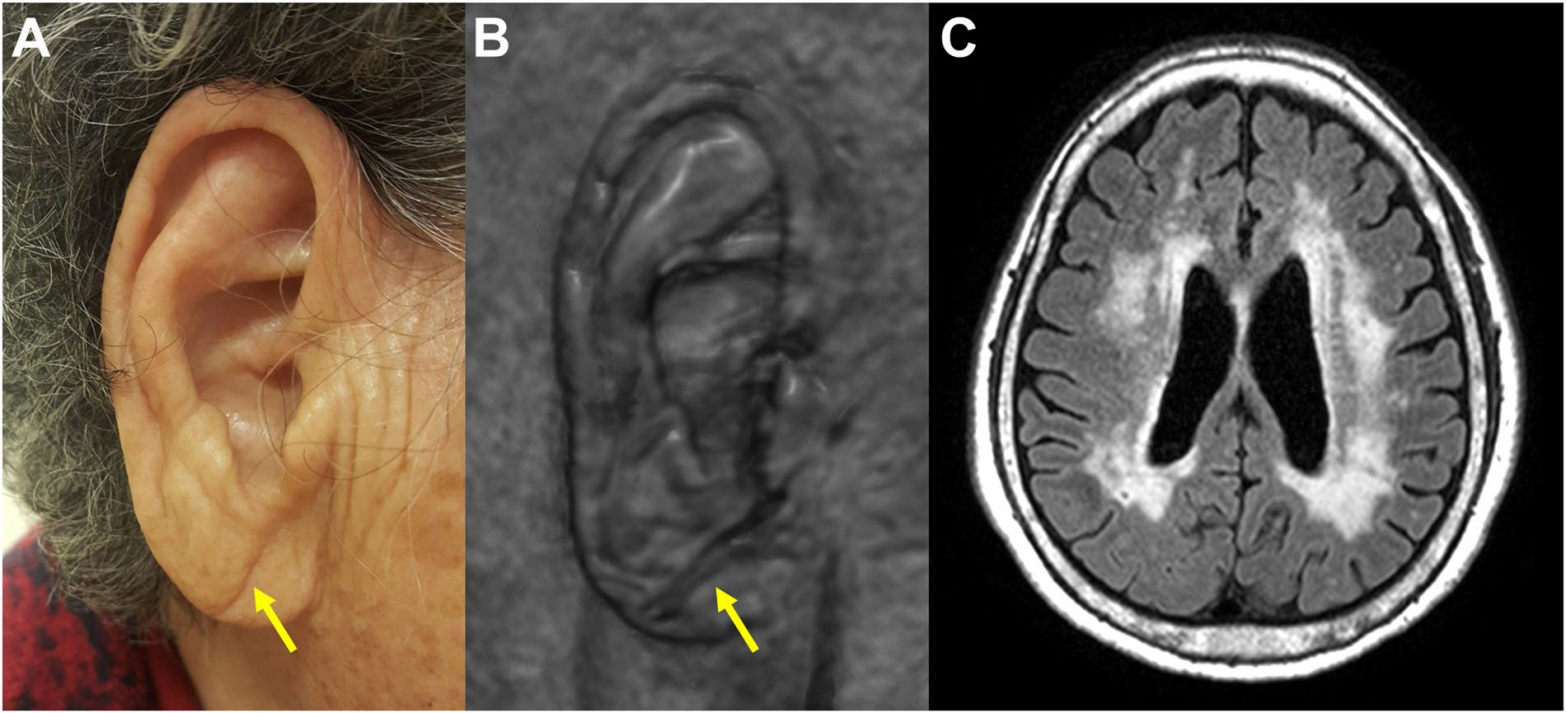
DELC (arrows) on photograph (A) and 3D-reconstructed MRI (B) in a cognitively impaired patient. The patient’s brain MRI shows severe WMH on FLAIR imaging (C). DELC = diagonal earlobe crease; MRI = magnetic resonance imaging; WMH = white matter hyperintensities; FLAIR = fluid-attenuated inversion recovery.
